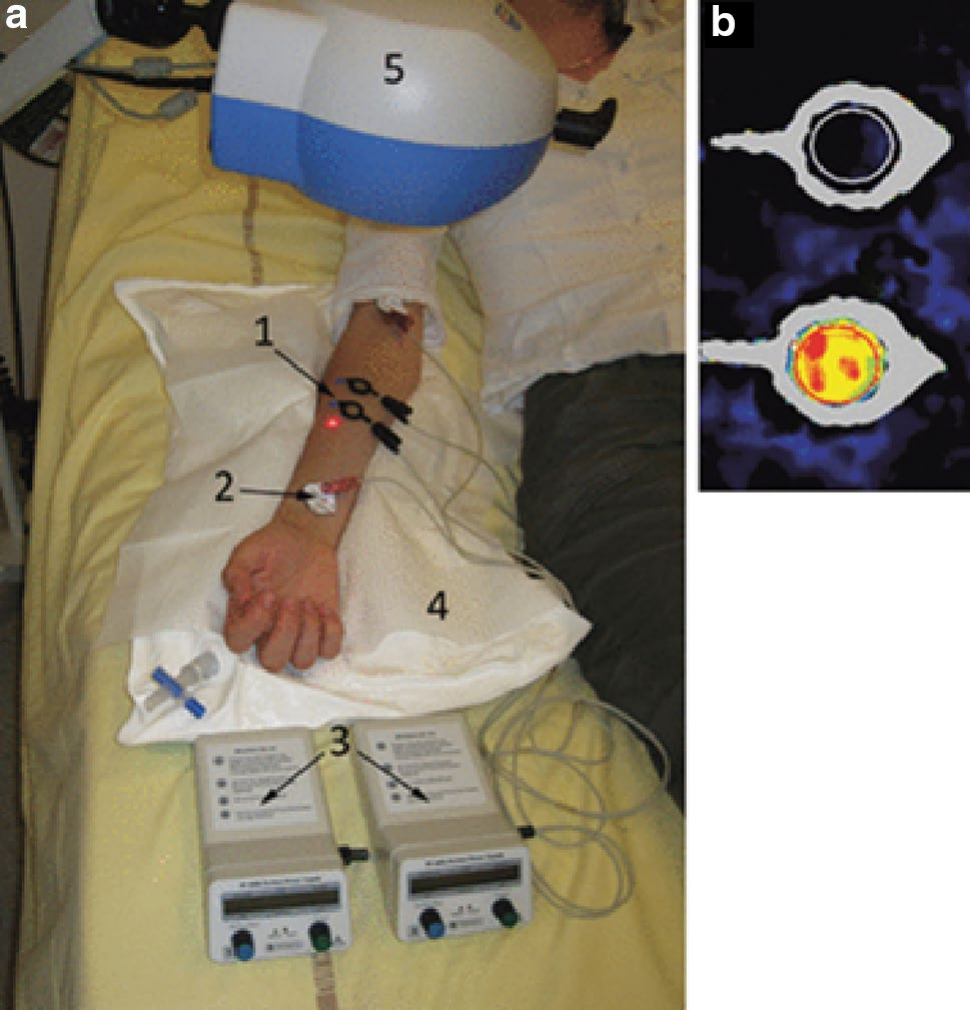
Example of an iontophoresis experimental setup. a Cathodal iontophoresis of a drug and control while recording skin blood flux with laser Doppler imaging (#5); (1) active electrode containing the drug, (2) passive electrode, (3) current generators connected to the electrodes, (4) vacuum cushion to reduce movement artefact. b Example of skin blood flux recorded during iontophoresis of sodium nitroprusside (bottom) and saline (top). With permission from Roustit and Cracowski (2012)
